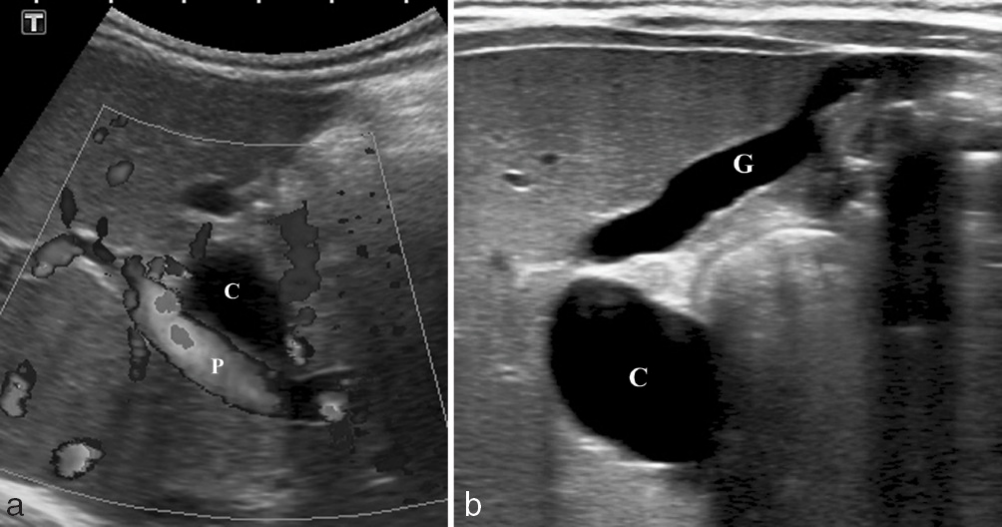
Ultrasound of the abdomen of case 2 showing (a) a large anechoic cyst (C), without colour Doppler signal, adjacent to the main portal vein (P). (b) The cyst (C) was connected to a gallbladder (G) with an abnormal appearance (small and elongated).
